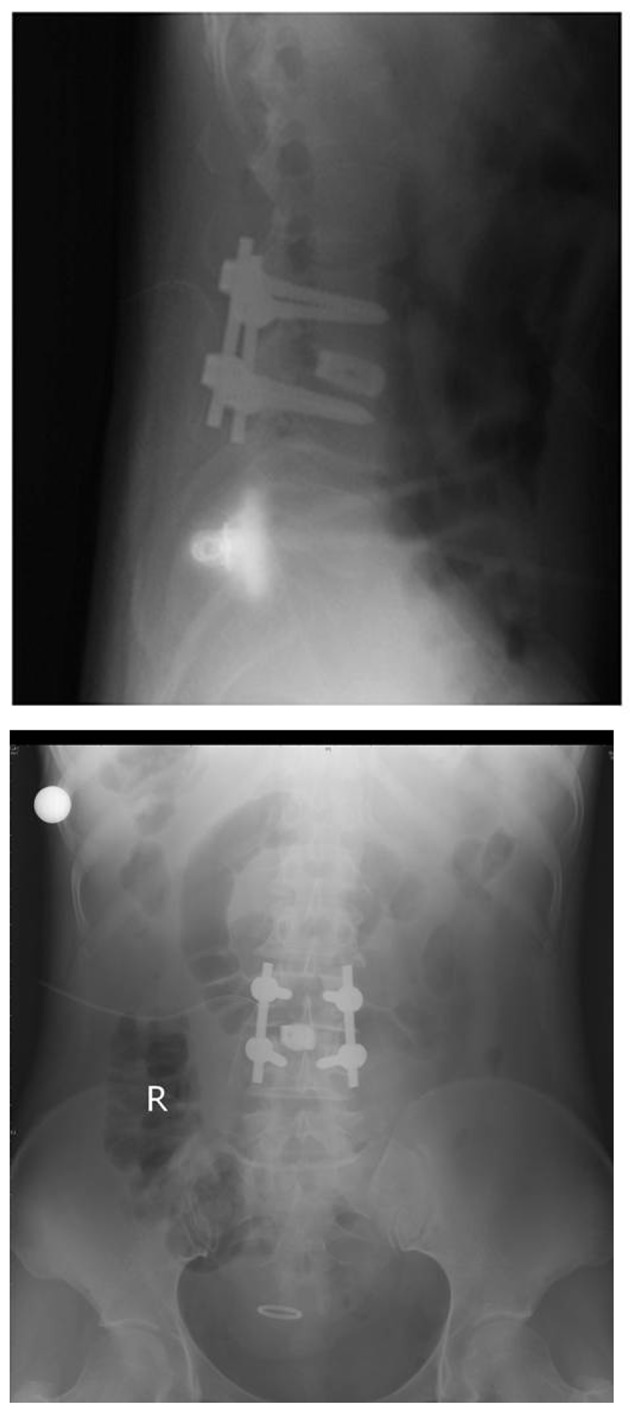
X-ray of the (A) lateral view and (B) AP view following minimally invasive transforaminal lumbar interbody fusion (MI-TLIF) surgery.
